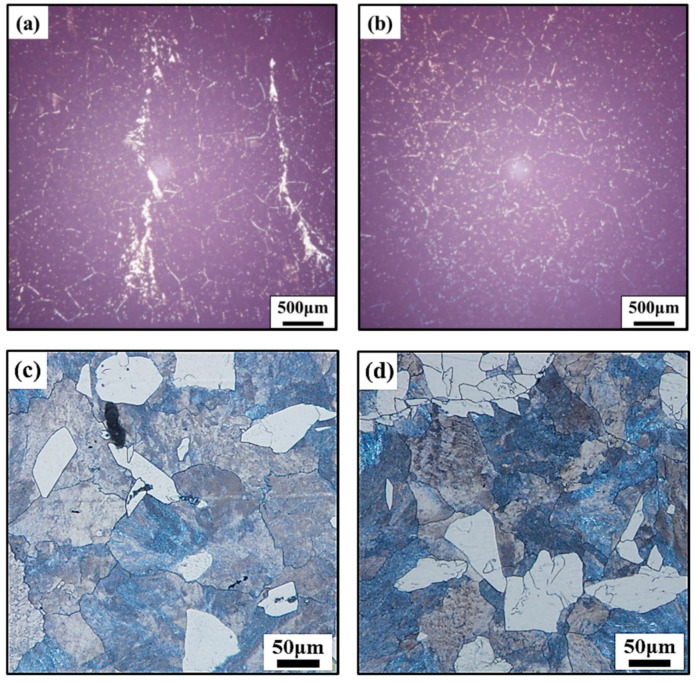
The SEM and OM images of 35CrMo steel ingot at 1/2R in Figure 1: (a,c) without ultrasonic, (b,d) with ultrasonic.
